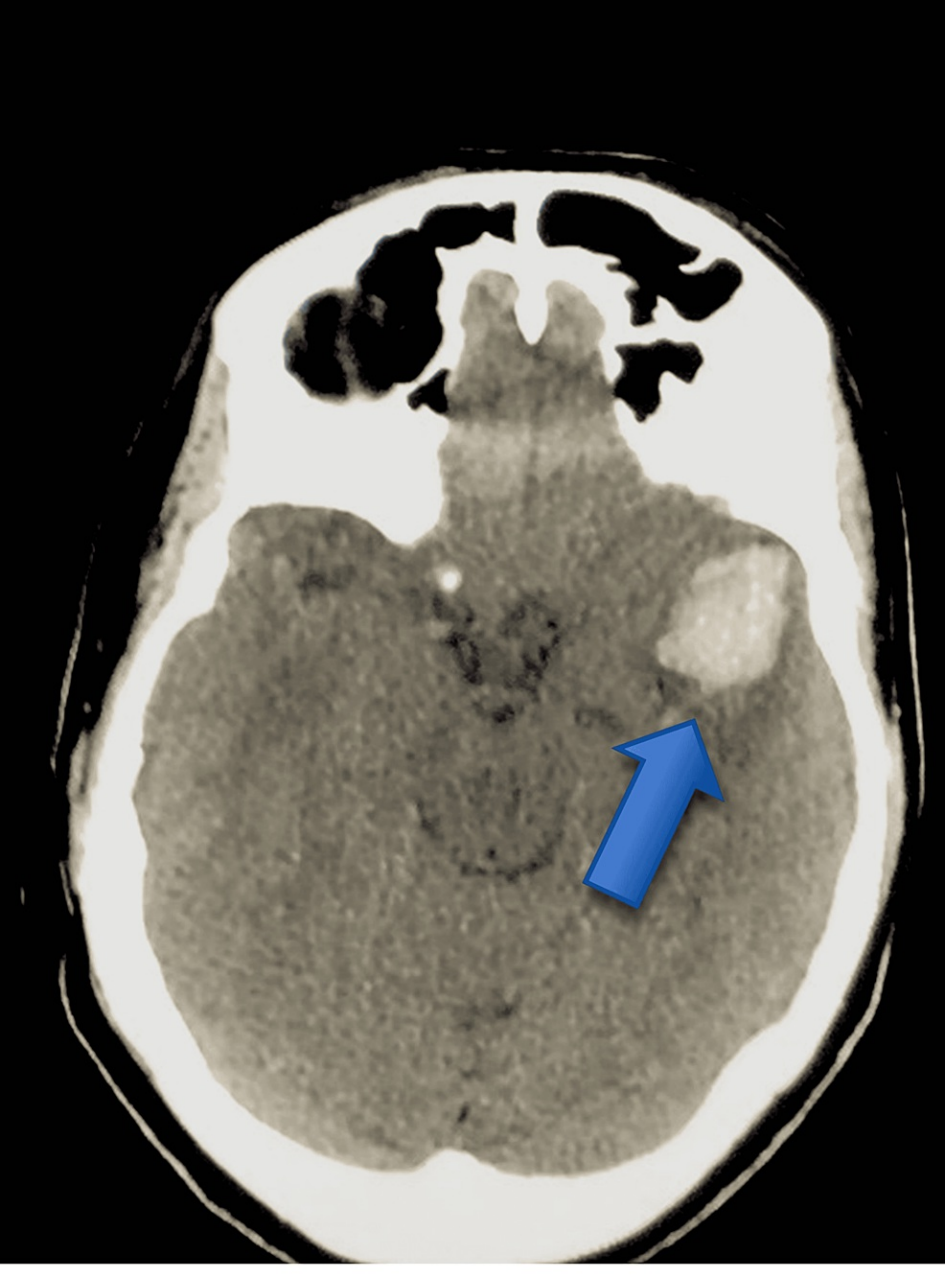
CT image of intraventricular hemorrhage in the left lateral ventricle. The arrow points to the 2.8 cm × 2.1 cm × 3.2 cm left temporal lobe hemorrhage with intraventricular blood in the left lateral ventricle and possibly temporal horn on the left and surrounding edema.
